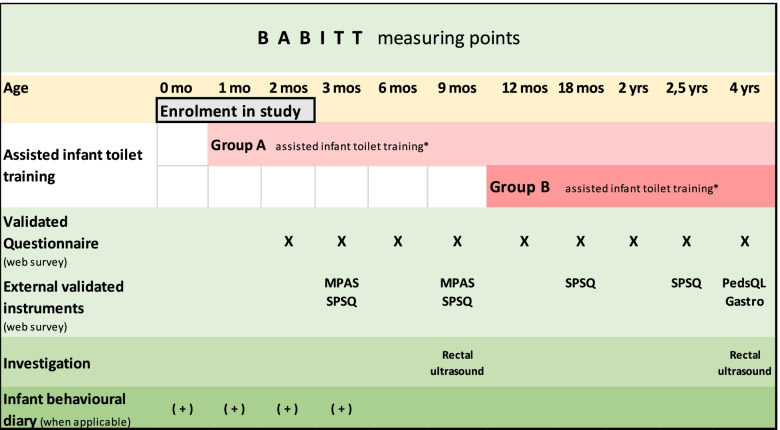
Time schedule over measuring points for the questionnaires (web surveys) (X) including the external validated instruments Maternal Postnatal Attachment Scale (MPAS), Swedish Parenthood Stress Questionnaire (SPSQ), Pediatric Quality of Life Inventory Gastrointestinal Symptoms Module (PedsQLGastro), and rectal ultrasound and infant behavioral diary (+) in the BABITT-study
